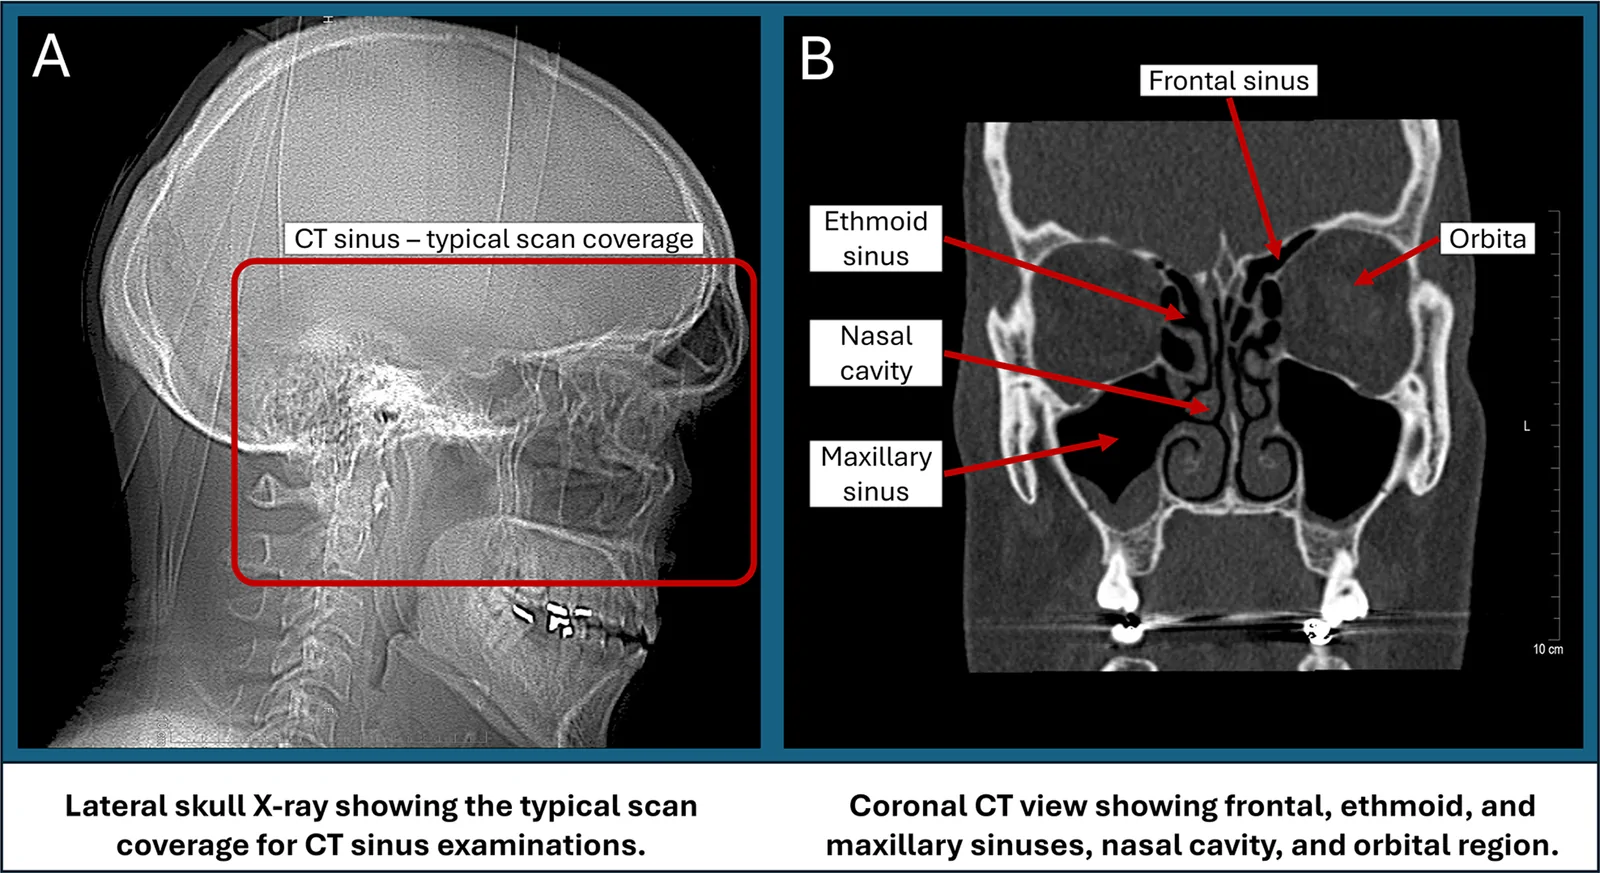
Computed tomography (CT) of the sinuses of a 54-year-old male. A is based on the Oslo University Hospital Procedure CT 5E1 Sinuses. 2025. Available from: https://ehandboken.ous-hf.no/document/14178
